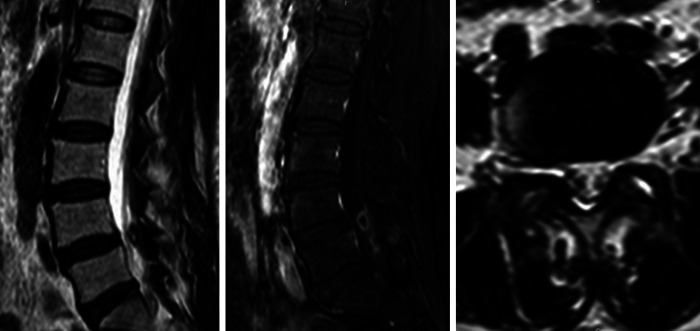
Preoperative MRI of the cyst lesion showing T2 hyperintensity with significant rim enhancement after administration of gadolinium (Gd).
